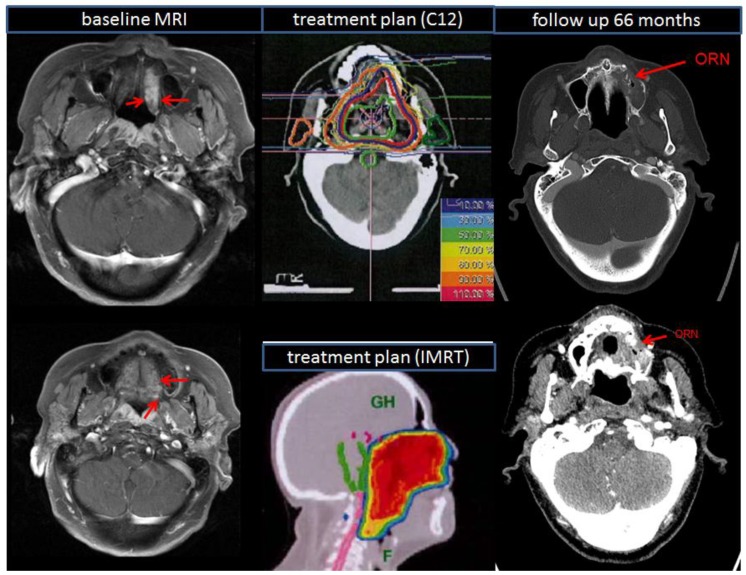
Radiation-induced osteoradionecrosis of the upper jaw: baseline MRI scan (left side) and follow-up CT scan (right side) of a patient who undergone surgery of adenoid cystic carcinoma of hard palate and additive bimodal radiotherapy. 66 months after RT, there was increased soft tissue as well as erosion of the posterior wall of the left side of maxilla. In comparison with the initially-treated radiation plan, the lesion occurred in an irradiated region of the hard palate at the edge of the 95%-isodose (middle up: carbon ion isodose plan alone, middle down: IMRT isodose plan alone). Abbreviations: radiotherapy (RT), computed tomography (CT), magnetic resonance imaging (MRI), intensity modulated radiotherapy (IMRT), carbon ions (C12).
